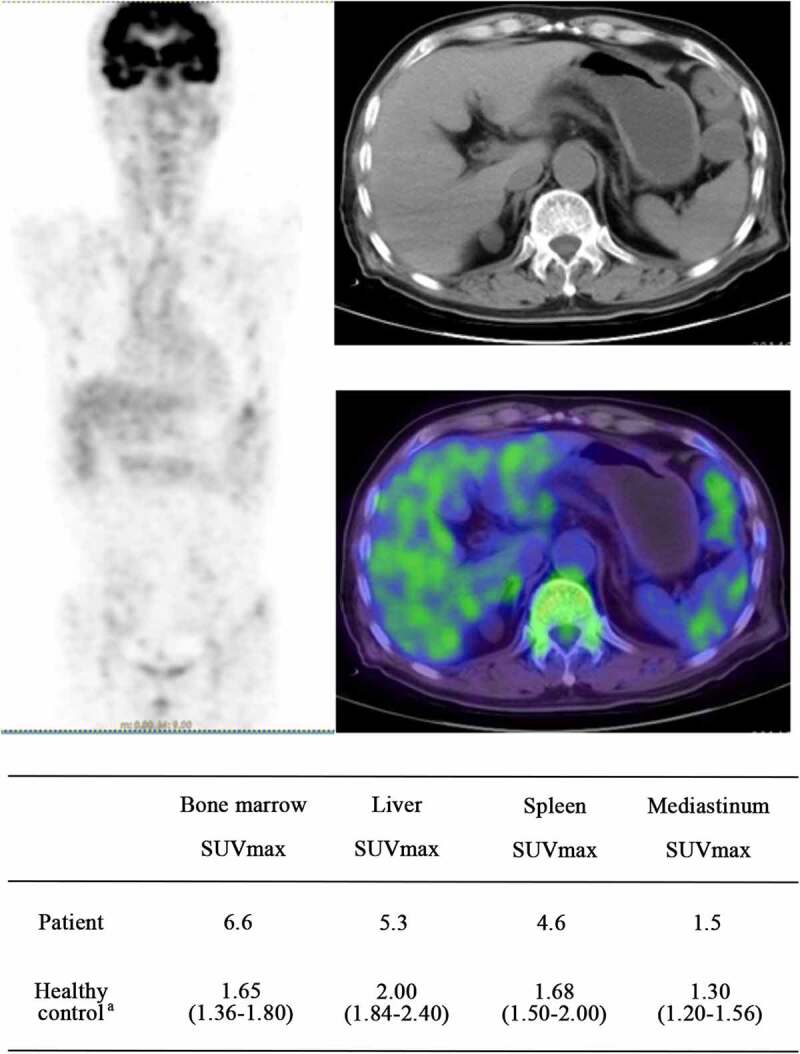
The images of whole body 18F-FDG-PET/CT. a:(median, quartile), SUVmax of healthy people who underwent 18F-FDG-PET/CT examination at our hospital; In the whole-body 18F-FDG-PET/CT images, the higher that the tissue uptake rate of FDG is, the higher that the SUV value is, and the darker that the color in the image is. As the SUV value rises, the image color changes from blue to green, yellow, or even red. Abbreviations: SUVmax, maximum standardized uptake value; 18F-FDG-PET/CT, 18F-fluorodeoxyglucose (18F-FDG) positron emission tomography and computed tomography (PET-CT)
